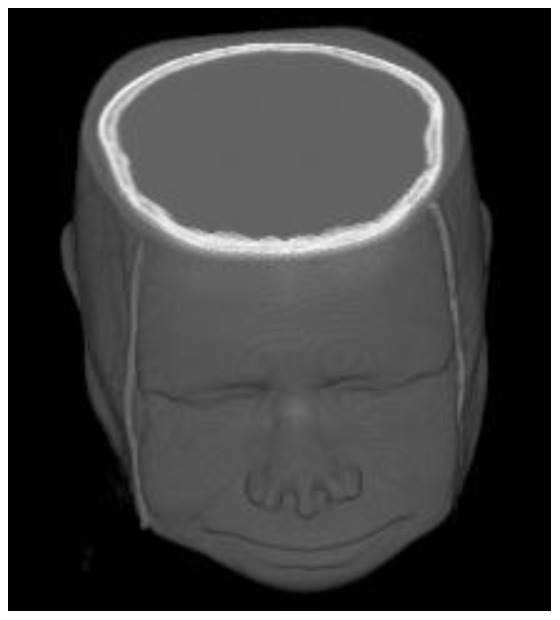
Head CT model approximated as an ellipse of semi-major axis
